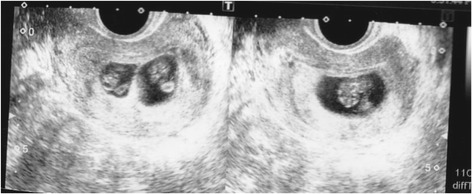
Ultrasound image of a dizygotic trichorionic triamniotic triplet in the seventh week of pregnancy
